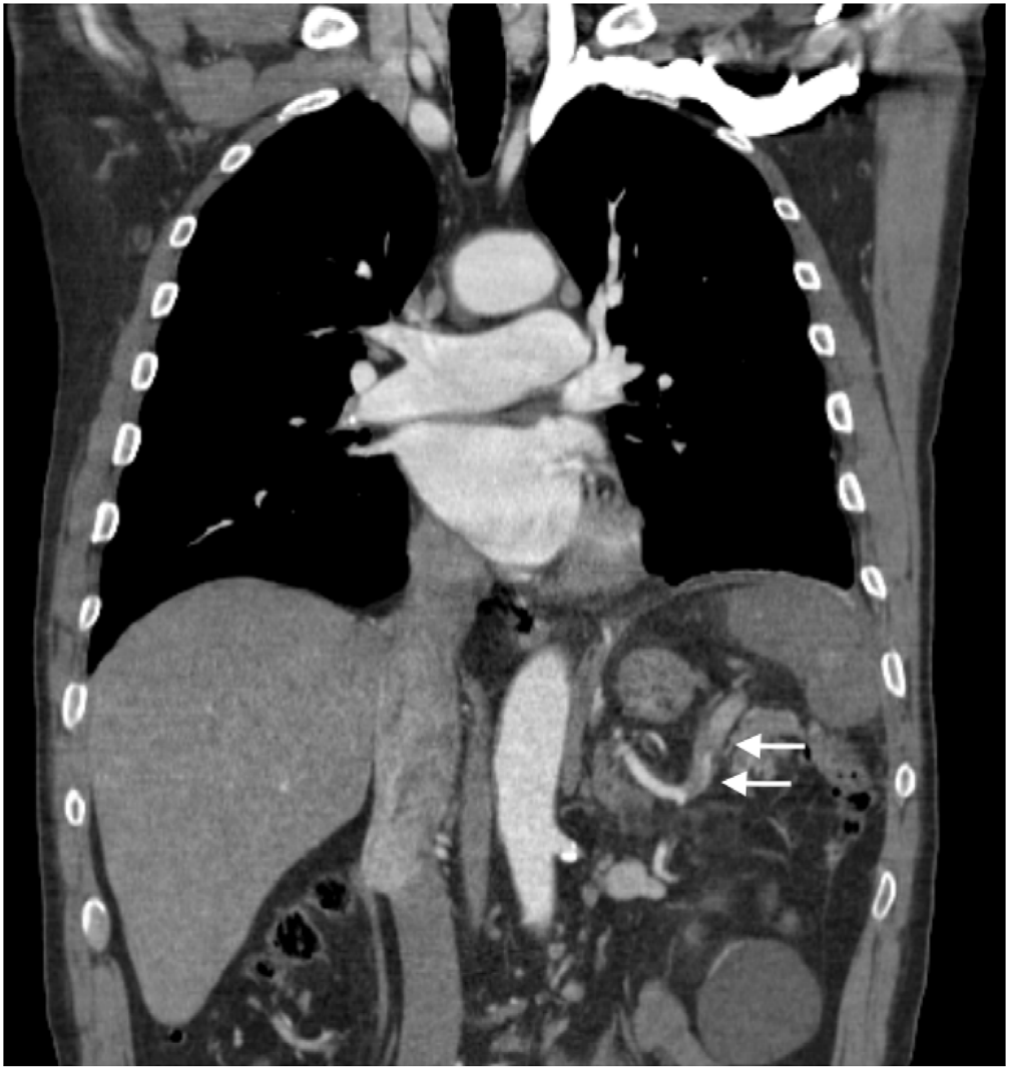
Coronal CTA image showing hypodense areas throughout the spleen consistent with splenic infarctions associated with a thrombus in the distal region of the splenic artery.
